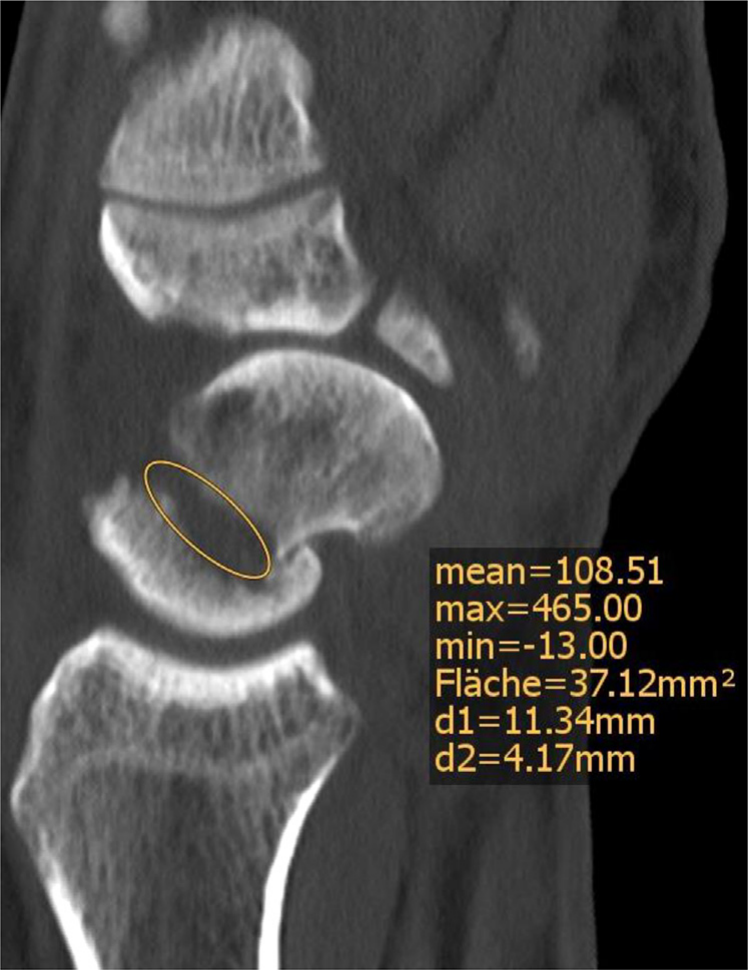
Measurement of the Defect zone sagittal view in a computed tomography and measurement of defect zone with an ellipse (mm^2^ = square millimetres).
